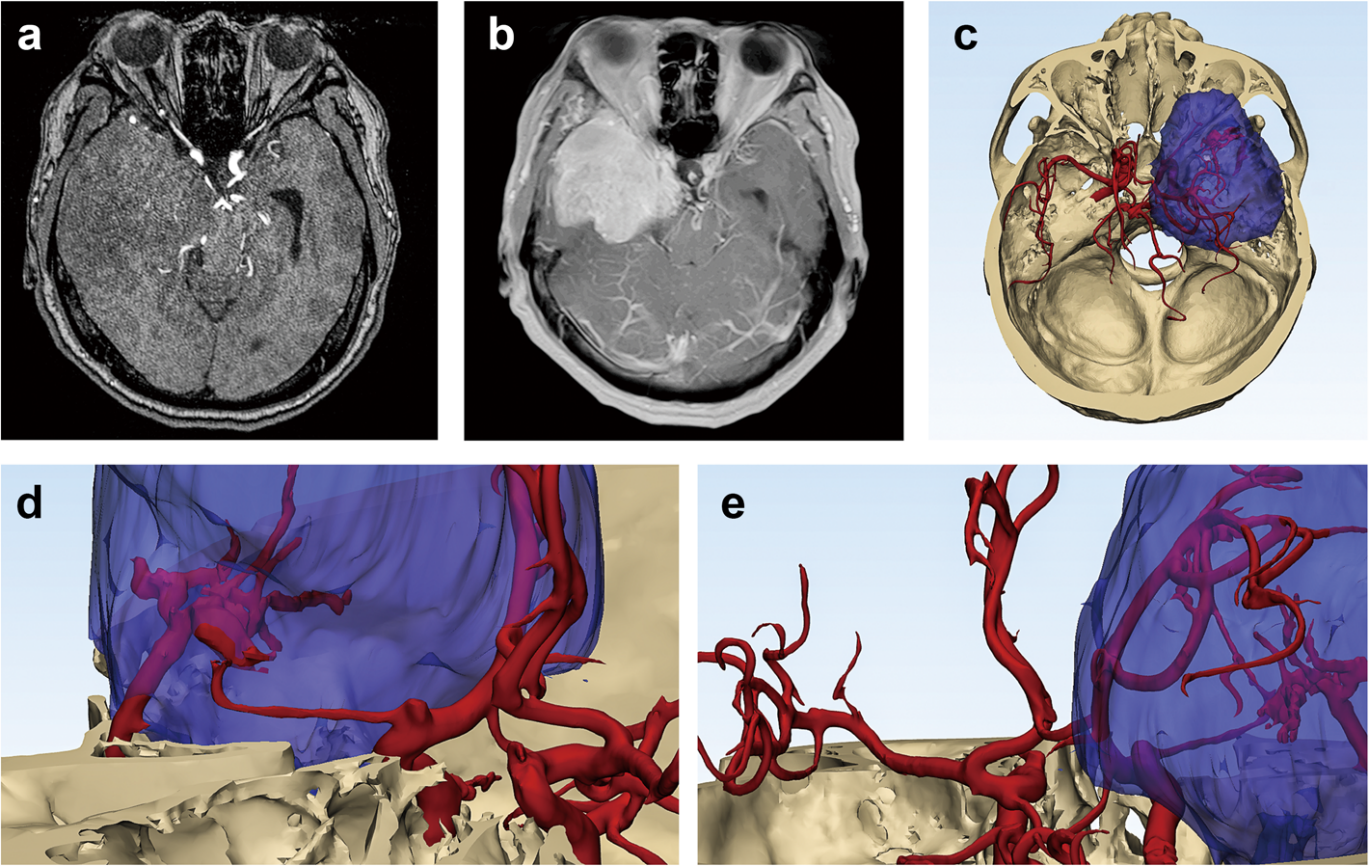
A patient who complained about the weakness of lower extremities for half a year. a Axial view on MRA showing the C4 segment of ICA and M1 segment of MCA displaced by the tumor. b Axial view on MRI showing a giant right medial sphenoid wing meningioma. c The 3D structures of brain tumor (purple), arteries (red), and bone structure (beige) reconstructed and imaged by Mimics software showing the tumor grew into the cavernous sinus and invaded the bone. d, e Enlarged view of the structures showing the tumor attached to the C4 segment of ICA and M1 segment of MCA and narrowed the lumen of M1
